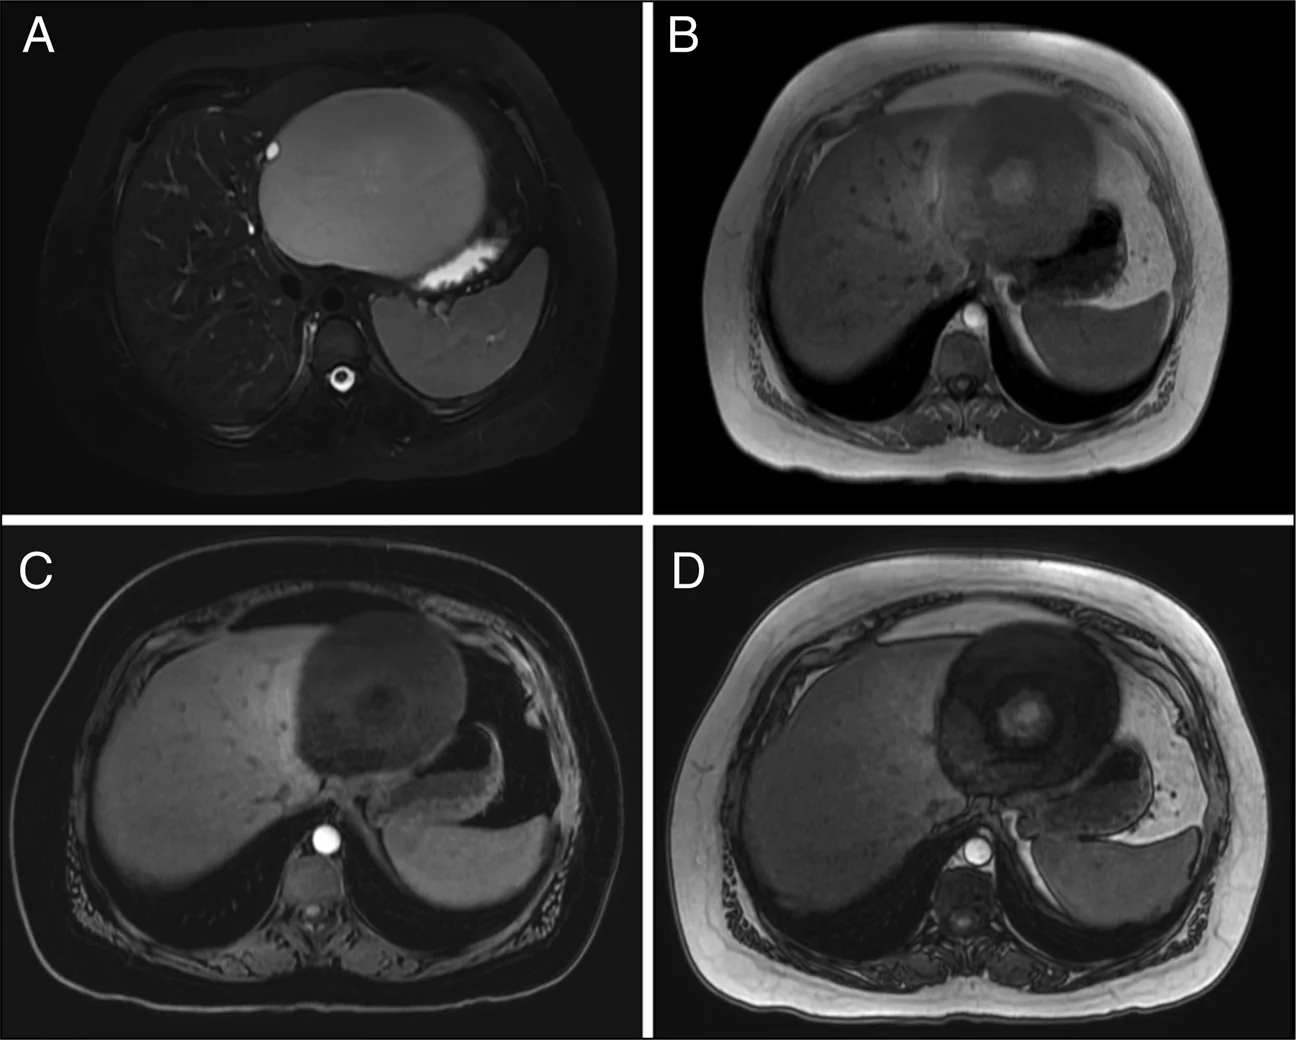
A 17.7 cm complicated cyst centered in the anterior upper abdomen alongside the left lobe of the liver. There is a smaller 11 mm mural cyst along its right superolateral wall (A), and in the nondependent upper portion, there is a 4.6 cm nodule (B–D). (A) Axial T2-weighted fat-suppressed MRI (navigation sequence). (B) Axial T1-weighted in-phase 3D LAVA precontrast breath-hold MRI. (C) Axial T1-weighted out-of-phase 3D LAVA precontrast breath-hold MRI. (D) Axial T1-weighted water-only 3D LAVA precontrast breath-hold MRI. MRI, magnetic resonance imaging.
